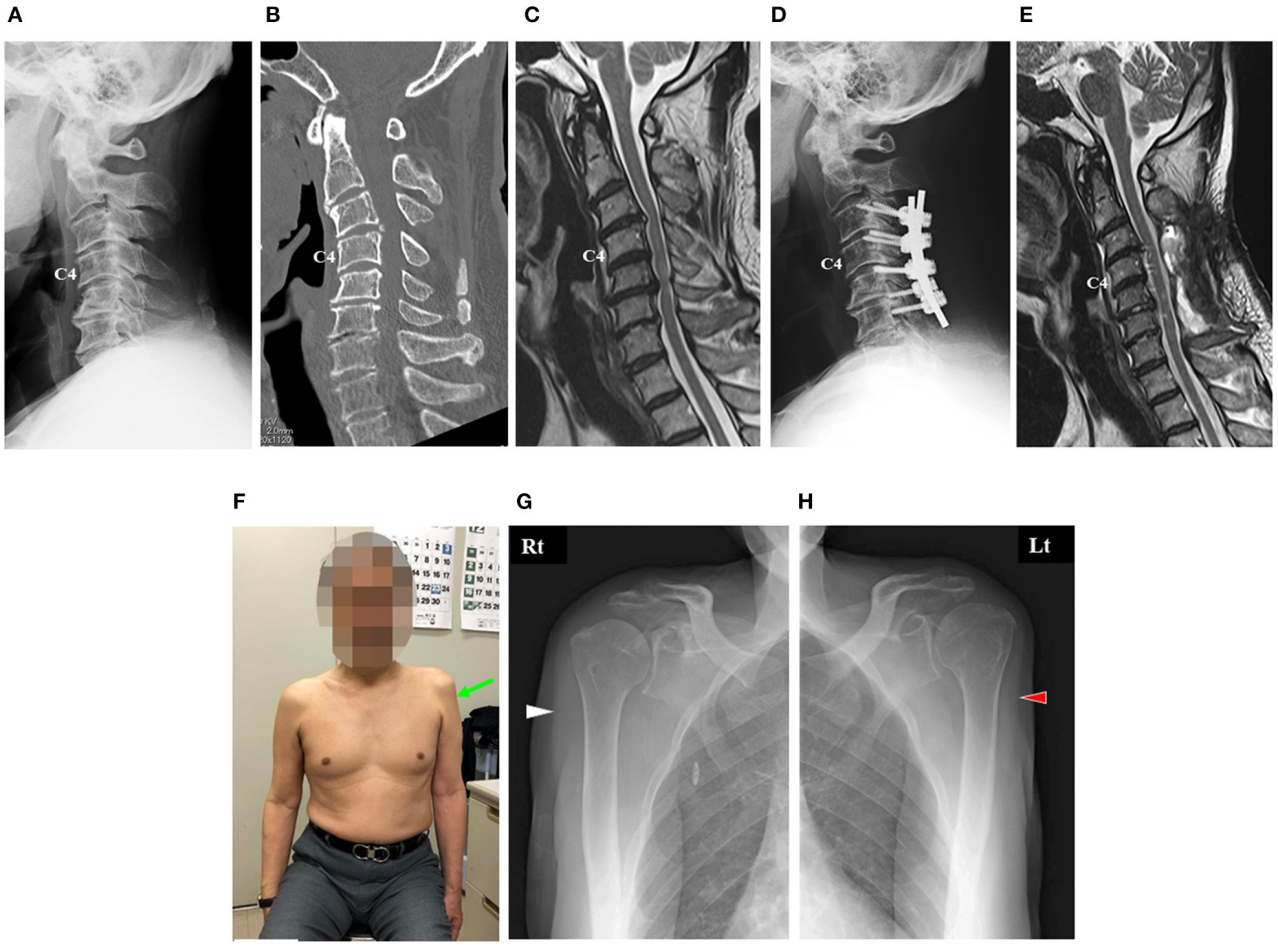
Imaging findings of the cervical spine of the patient before surgery (A–C). Image findings of his cervical spine after surgery (D,E). Photograph and radiographs of the shoulders of the patient 7 months after surgery (F–H). (A) Cervical lateral radiograph revealing slight kyphosis of his cervical spine. (B) Sagittal reconstruction computed tomography (CT) image revealing disc space narrowing with a posterior spur at C5–C6 and C6–C7 and postdiscal ossification at C3–C4. (C) Midsagittal T2-weighted magnetic resonance (MR) image revealing that the spinal cord was severely compressed anteriorly at C3–C4 and C5–C6. (D) Cervical lateral radiograph 2 weeks after surgery revealing instrumented fusion at C3–C6. (E) Midsagittal T2-weighted MR image 4 weeks after surgery revealing adequate decompression of the spinal cord. (F) Anterior view showing muscle atrophy around his left shoulder. (G,H) Anterior–posterior radiographs of his right shoulder (B) and left shoulder (C) showing marked muscle atrophy in his left deltoid (red arrowhead) compared with that in his right deltoid (white arrowhead).
